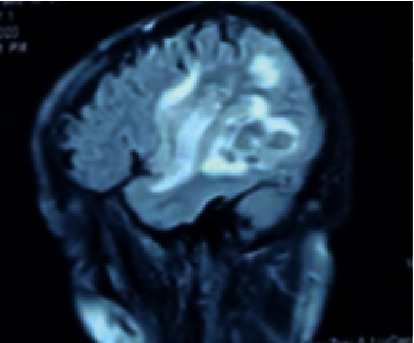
Brain MRI (FLAIR) in the sagittal plane showing hyperintensity in the right temporal lobe.
